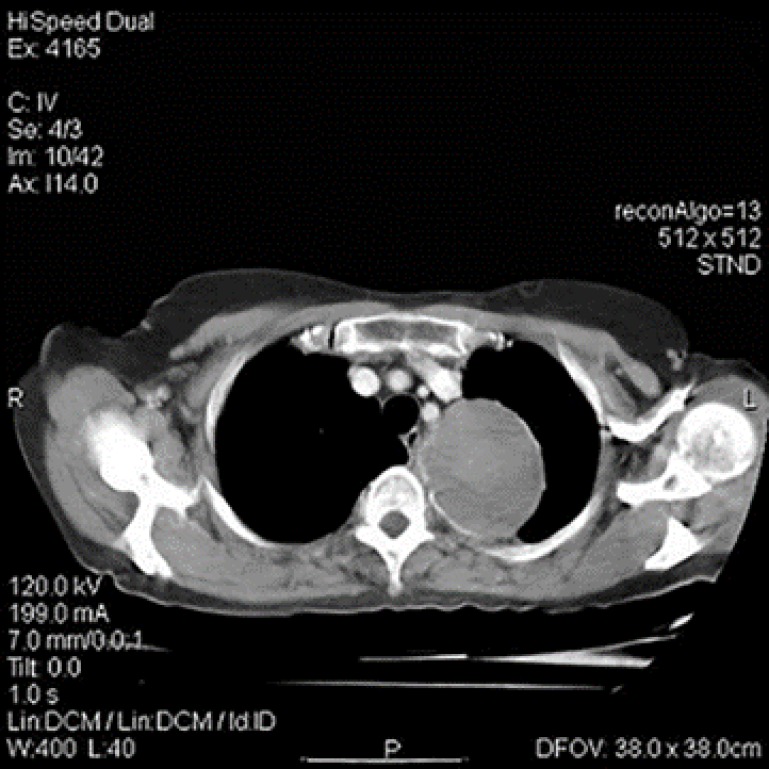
Axial computed tomography showing a large round heterogenous mass with smooth border at mediastinal boarder of the left hemithorax
